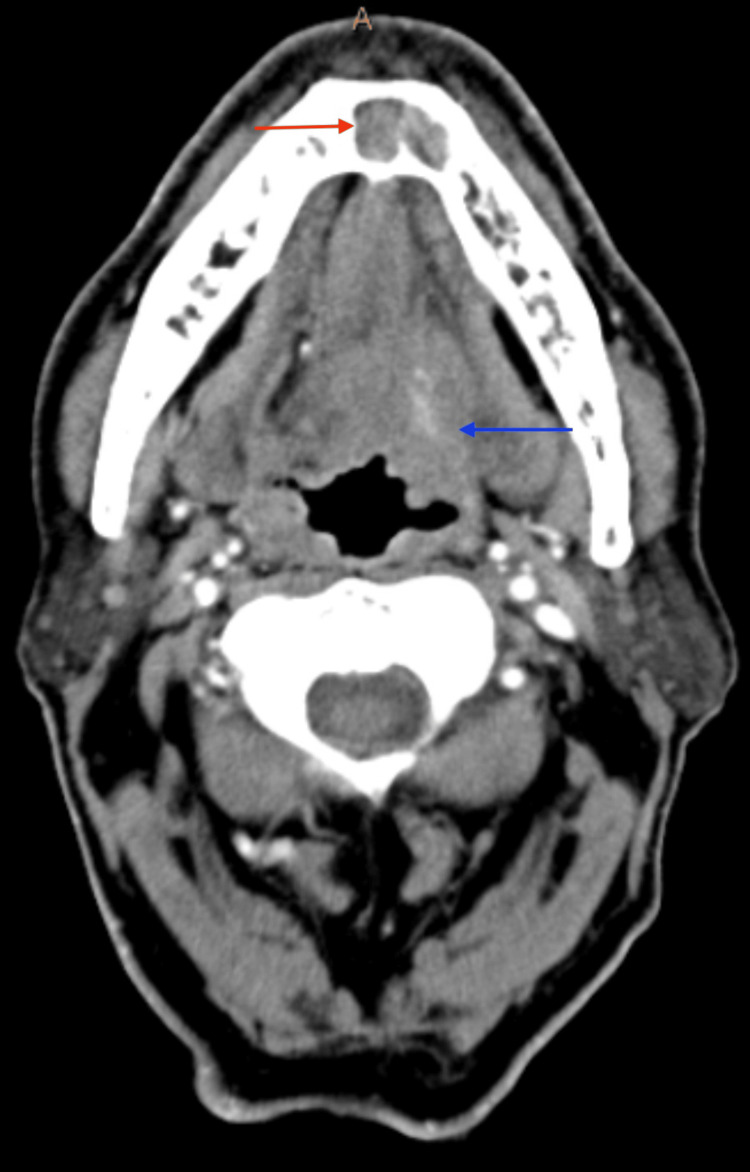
Axial CT Neck of Mandibular and Base of Tongue Mass Axial CT neck with contrast showing a soft tissue mass in the mandibular symphysis (red arrow) and a left base of tongue mass (blue arrow)
